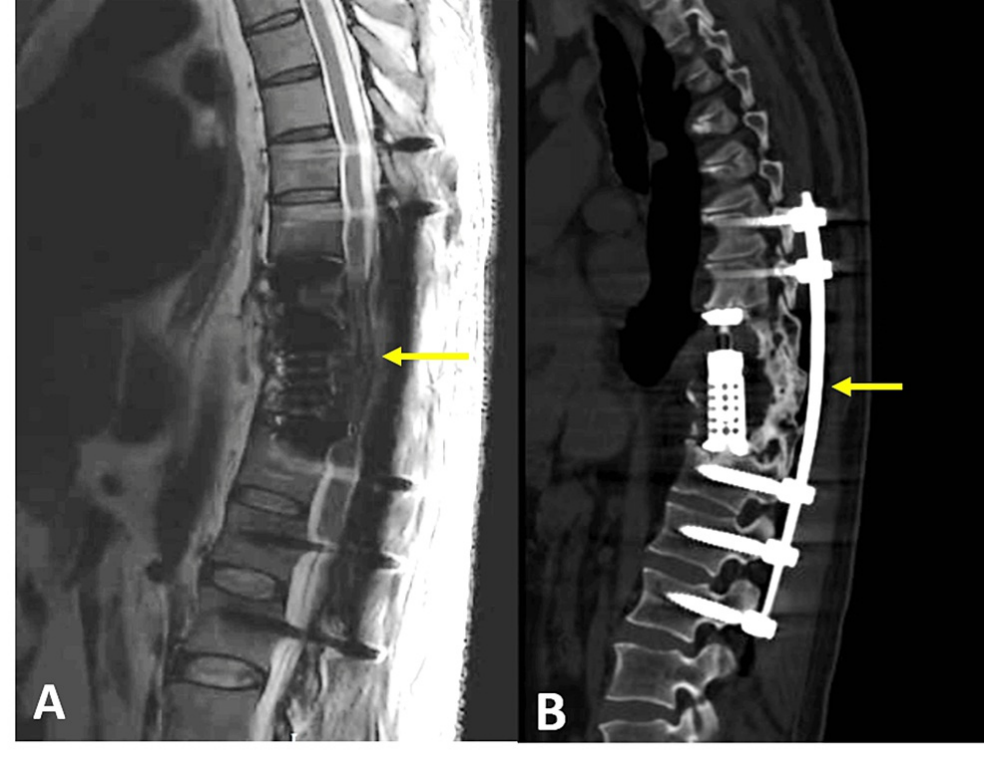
Postoperative imaging studies. (A) Sagittal T2 magnetic resonance imaging with adequate spinal decompression and restoration of thoracic kyphosis and vertebral height (yellow arrow); (B) Sagittal computed tomography showing adequate placement of the transpedicular screws and expandable cage (yellow arrow).
